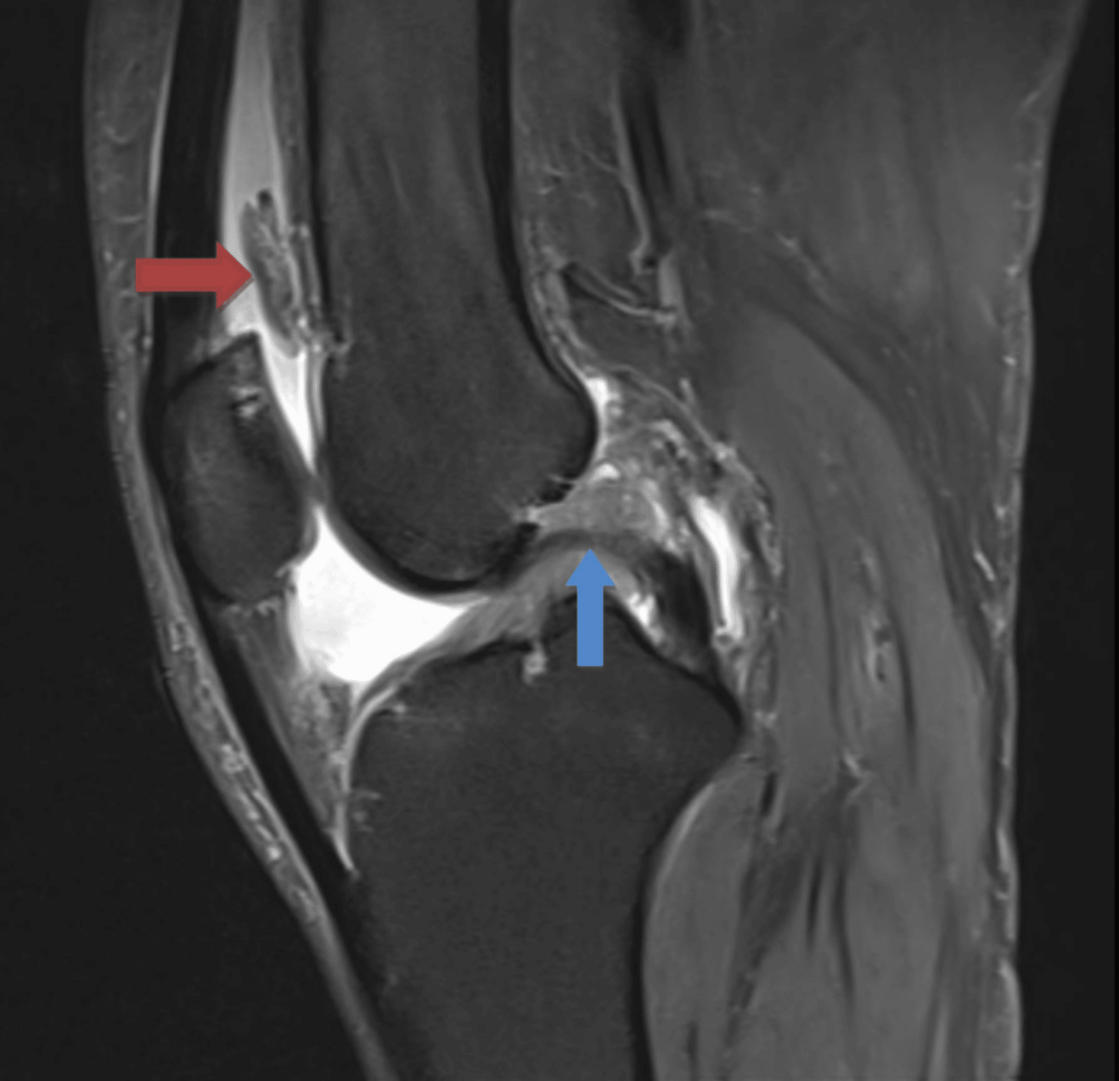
Sagittal section of the MRI of the right knee Red arrow: Frond-like mass arising from the suprapatellar pouch, Blue arrow: Mucoid degeneration of the ACL ACL: Anterior cruciate ligament
